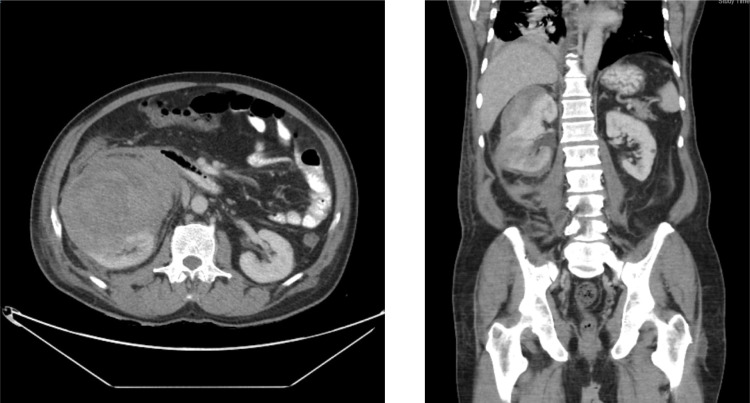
A CT scan of the abdomen and pelvis shows 10 × 11.2 × 18.7 cm right large subcapsular, perirenal and retroperitoneal haematoma compressing and exerting mass effect on the kidney with a cortical defect at the lower pole with adjacent 2 cm dense foci.
